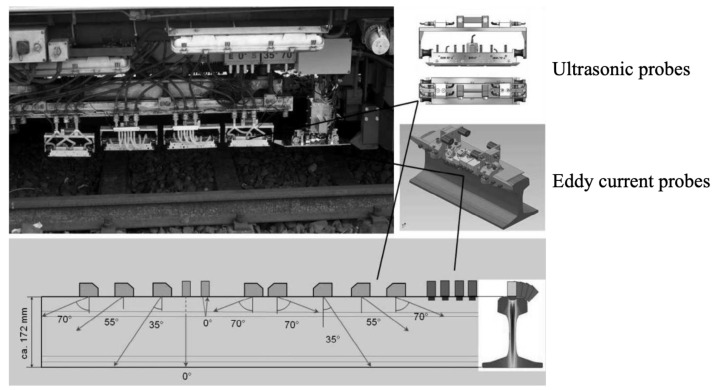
Probe arrangement of ultrasonic and eddy current integrated system [78].
